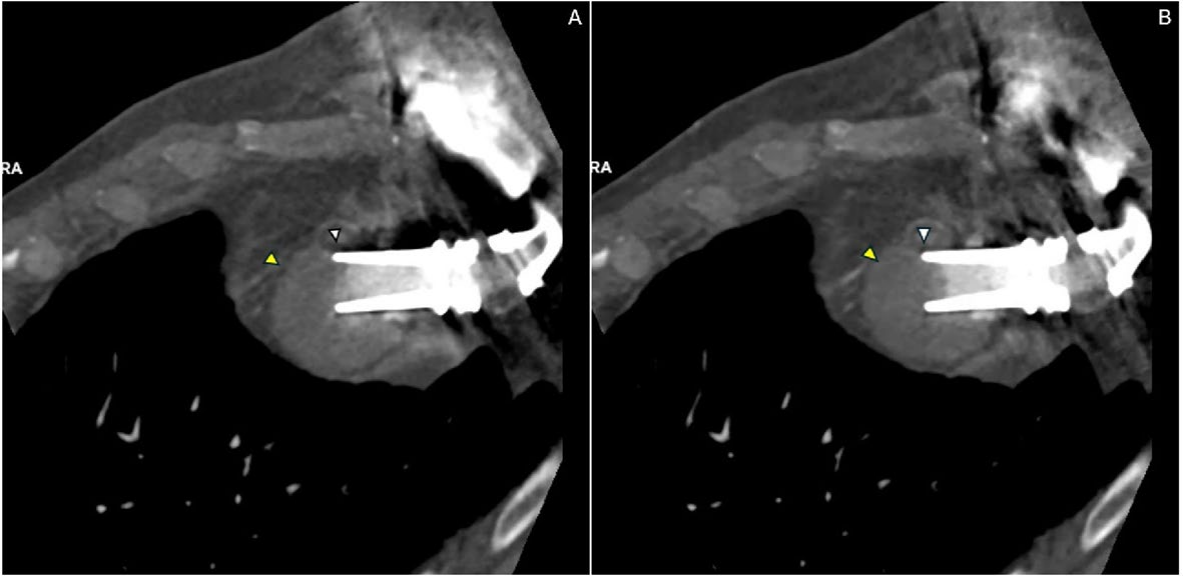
Long-axis multiphasic cardiac computed tomography angiography (CTA) demonstrating dynamic left ventricular assist device (LVAD) inflow cannula obstruction. A Diastolic phase multiplanar reformatted cardiac CTA image reconstructed along the long axis of the LVAD inflow cannula demonstrates partial inflow obstruction as the apical interventricular septum abuts the cannula orifice (arrowhead). The inflow cannula orifice is identified by the arrow. This orientation, with the cannula directed toward the interventricular septum rather than parallel to the mitral valve annulus, predisposes to dynamic inflow obstruction. B Systolic phase image reconstructed at the same slice level demonstrates progression to complete inflow obstruction as septal myocardium is drawn further into the cannula orifice (arrowhead). The cannula orifice is again identified by the arrow, demonstrating phase-dependent dynamic inflow cannula obstruction (“suck-down”). Images represent matched reconstructions from the same cardiac cycle and slice position to allow direct phase comparison
